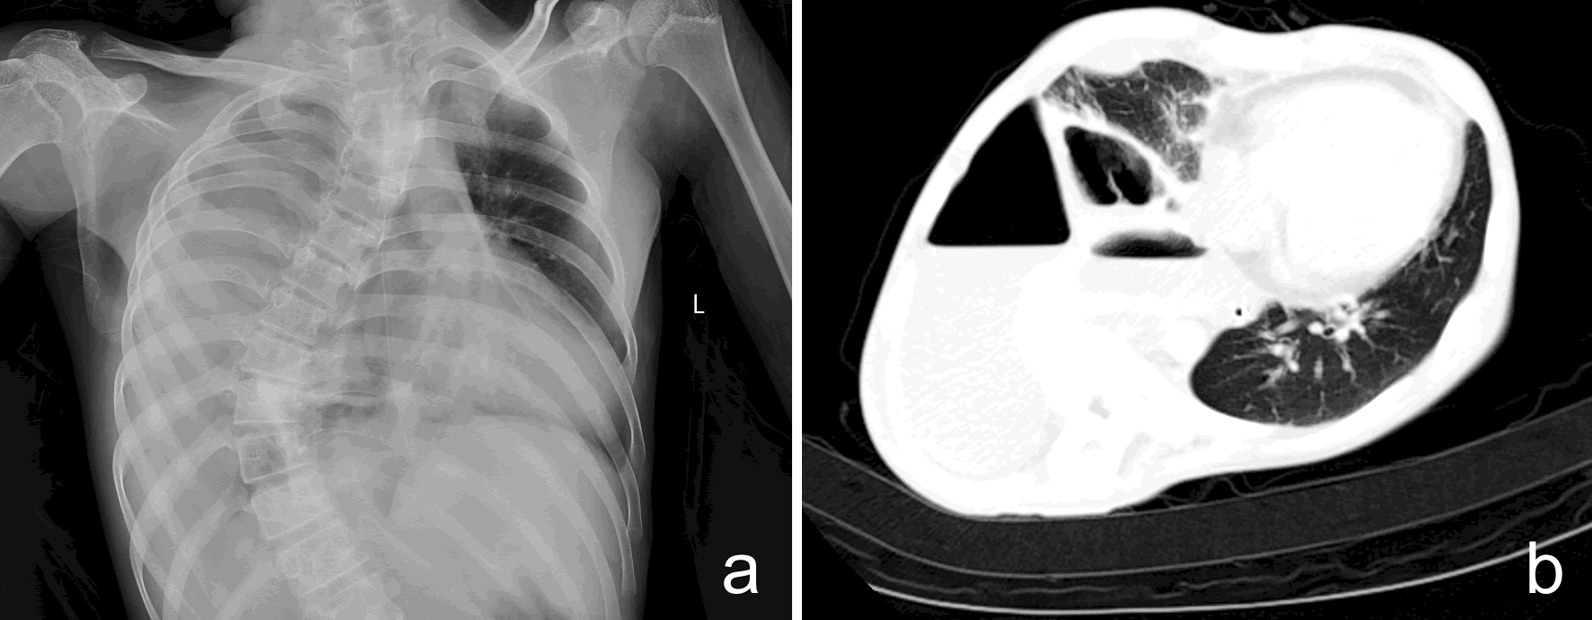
a Chest radiography on admission, showing massive right pleural effusion with blurred left heart border. b Chest CT at 2 days after admission, showing bilateral pneumonia, a large pyopneumothorax in the right pleural cavity, and an oblique fissure hydrothorax on the left
